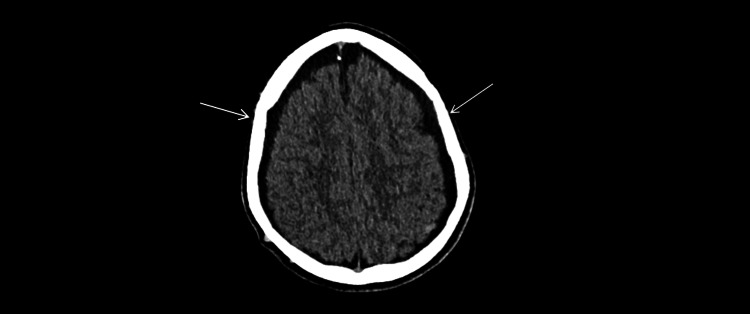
Non-contrast head CT (axial, brain window; W80/L40; 5-mm slices). Thin crescentic subdural fluid collections along the bilateral frontoparietal convexities (arrows), compatible with subdural hygromas.
